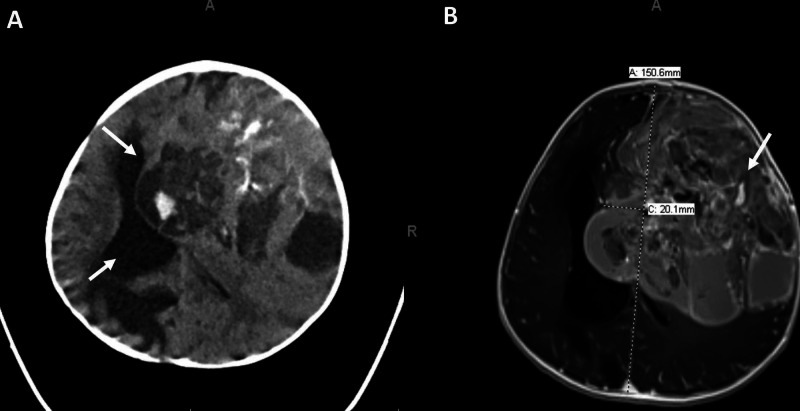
Diagnostic pre-resection imaging Diagnostic pre-resection imaging showing a frontotemporal heterogeneous and locally invasive mass causing obstructive hydrocephalous (arrow), 2 cm of midline shift (arrow), internal hemorrhage (arrow), and likely bony involvement of the left frontal bone. (A) Axial CT. (B) Axial MRI
